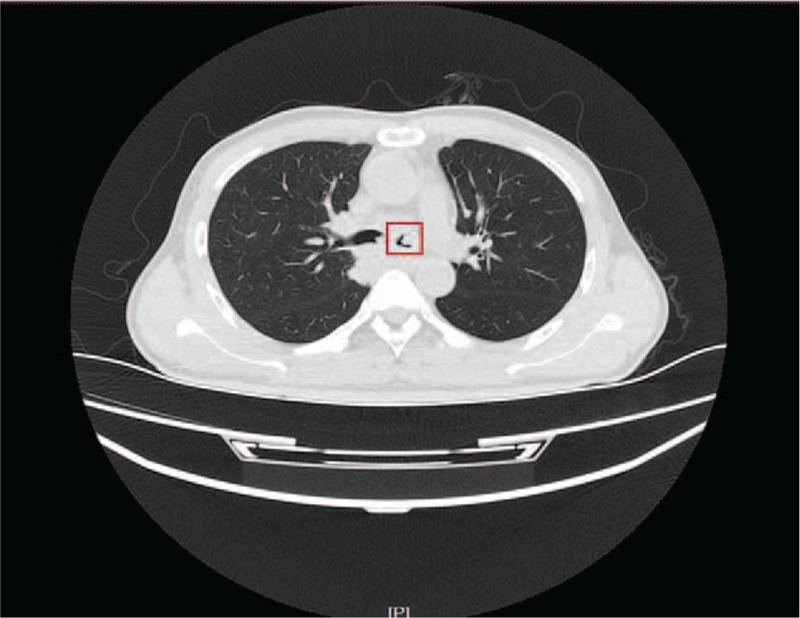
Chest CT on left main bronchus level before operation. CT = computed tomography.
